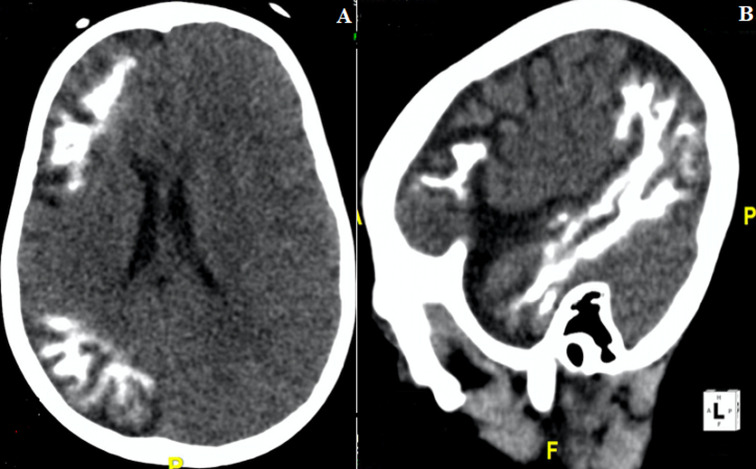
non contrast brain CT A) axial section showing a right hemi-cerebellar atrophy associated with gyriform fronto-parietal calcifications; B) right sagittal reconstruction showing these calcifications at the temporal lobe also
